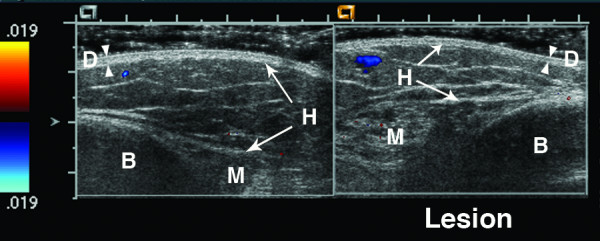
15 year old girl with linear scleroderma of her left leg that has progressed over the past three years. Her calf lesion shows erythema, warmth, and continuing tissue loss. Ultrasound shows an increase in lesion hypodermal vascularity; there are a few small color Doppler signals in both the lesion and normal hypodermis, and one large color Doppler signal in the lesion hypodermis (hypodermal vascularity = 1). D = dermis, H = hypodermis. Arrowheads indicate boundaries of dermis, while arrows indicate boundaries of hypodermis.
